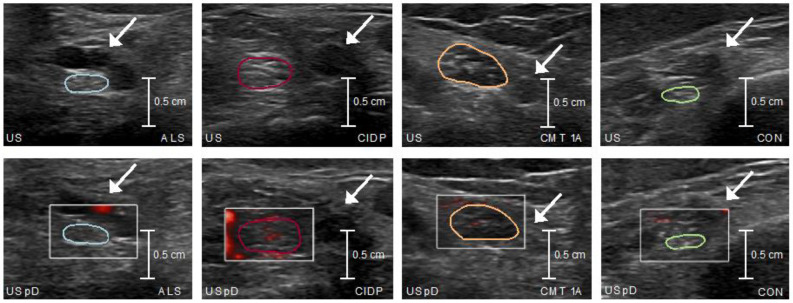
Ultrasound and ultrasound power Doppler—tibial nerve. (Top row) Representative B-mode ultrasound image of each subgroup. CSA of the tibial nerve is massively larger in the CMT (orange) and the CIDP (red) than in the ALS (blue) or the control (green) patients. (Bottom row) Microvascular blood flow at the same locations as in the top row images. CIDP (red) shows strengthened microvascular blood flow with the most red spots compared to ALS (blue), CMT (orange), and control (green). Color-coded tibial nerve cross-sectional areas; arrows indicate posterior tibial artery. US, ultrasound; US pD, ultrasound power Doppler; ALS, amyotrophic lateral sclerosis, CIDP, chronic inflammatory demyelinating polyradiculopathy, CMT, Charcot–Marie–Tooth disease; CON, controls.
